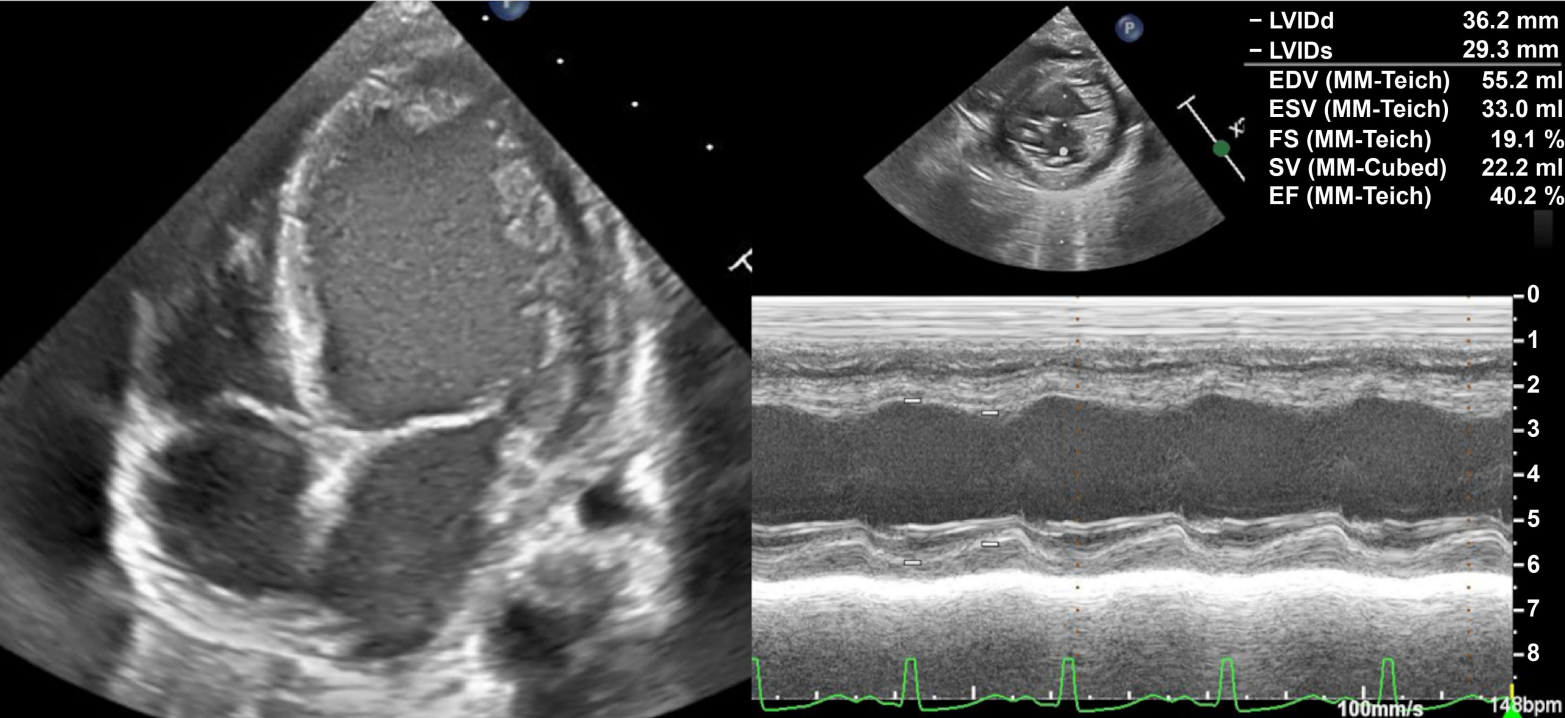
Admission echocardiography showed left ventricular enlargement and decreased contractility.
